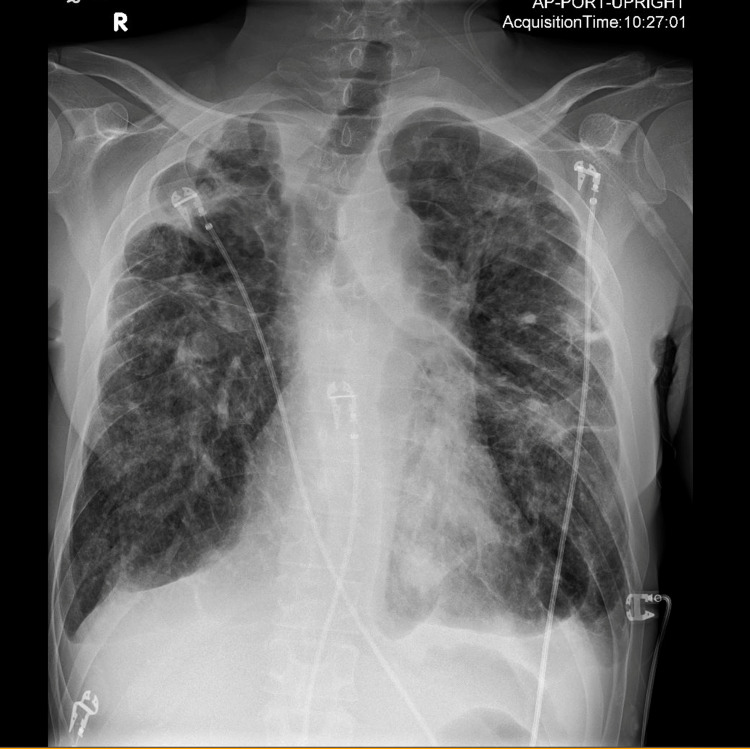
Chest X-ray, anterior-posterior view, demonstrating diffuse emphysematous and fibrotic changes consistent with advanced sarcoidosis
